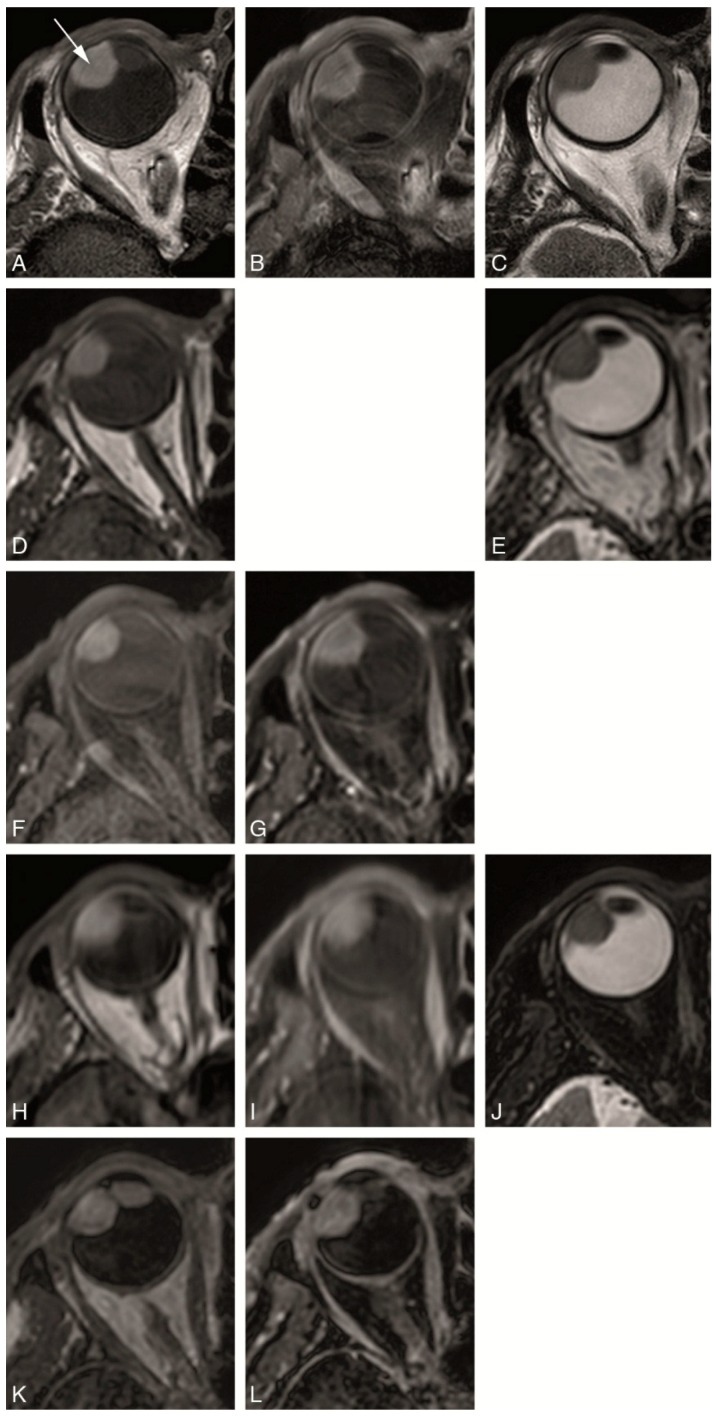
(A–L) Study protocol: Multiple anatomical axial MRI sequences in a patient with a uveal melanoma of the right eye (arrow). (A) MS 2 mm T1. (B) MS 2 mm enhanced T1 with fat signal suppression. (C) MS 2 mm T2. (D) MS 1 mm T1. (E) MS 1 mm T2. (F) MS 1 mm T1 with fat signal suppression. (G) MS 1 mm enhanced T1 with fat signal suppression. (H) 3D TSE 1 mm T1. (I) 3D TSE 1 mm enhanced T1 with fat signal suppression. (J) 3D TSE 0.8 mm T2 with fat signal suppression. (K) 3D TFE 0.8 mm T1. (L) 3D TFE PROSET 0.8 mm enhanced T1 with fat signal suppression.
